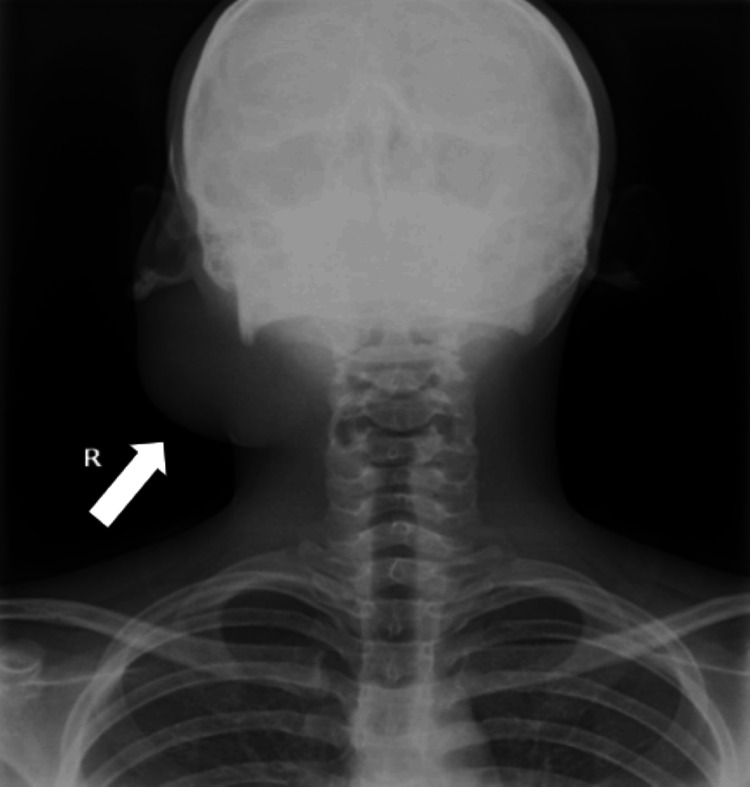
X-ray of the patient showing the swelling near the right infra-auricular region.
